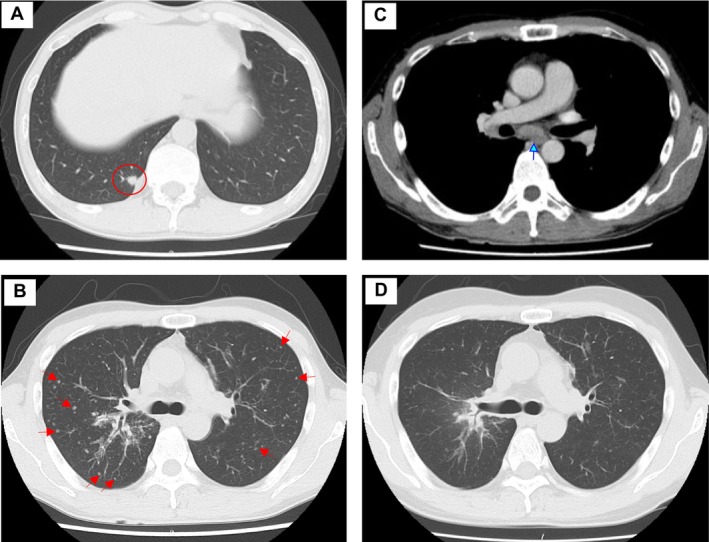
Chest computed tomography (CT) findings. CT revealed nodules in the right lower lobe (A) and miliary shadows in both lungs (B). The red circle denotes the primary lung tumor; red arrows indicate minimal intrapulmonary metastases, and the blue arrow highlights enlarged subcarinal lymph nodes. Contrast‐enhanced chest CT demonstrated enlarged subcarinal lymph nodes (C). After 11 months, the tumor showed a partial but stable response (D).
